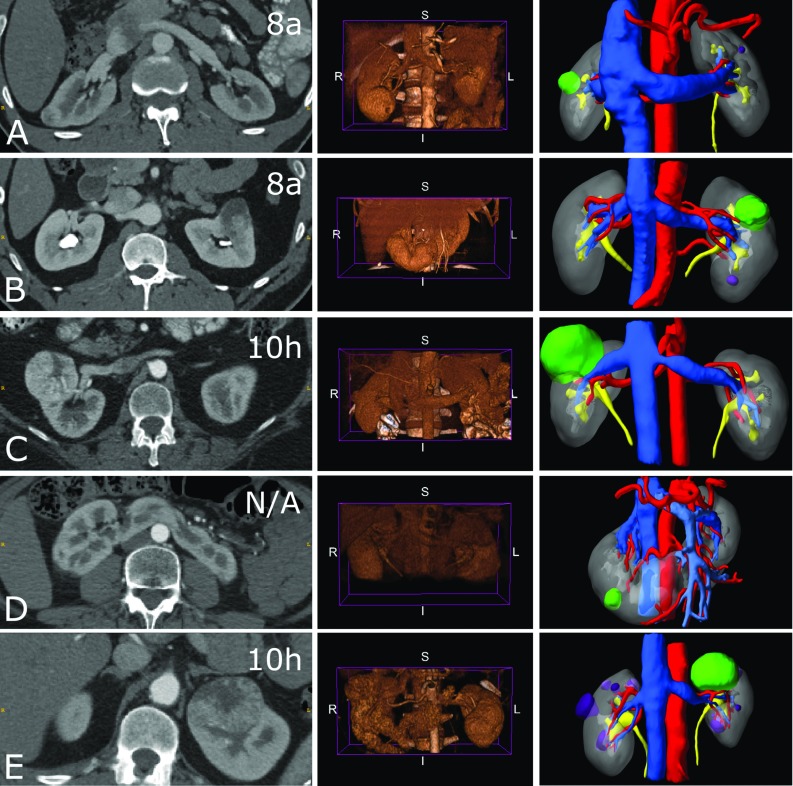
Representative imaging available for the 5 study cases a–e (rows) featuring: (left) arterial phase CT axial slice with the case RENAL score indicated in the top right corner. The horseshoe kidney case has not been given a RENAL score. (Middle) Arterial phase abdominal CT volume-rendered images (VRI) from an anterior viewpoint. (Right) A static screenshot of the generated, case-specific, interactive virtual 3D model which featured in the intervention arm of this study. The structure—colour keys are: artery—red; venous—dark blue; portal venous—light blue; tumour—green; cyst—purple; excretory—yellow; normal kidney—grey
